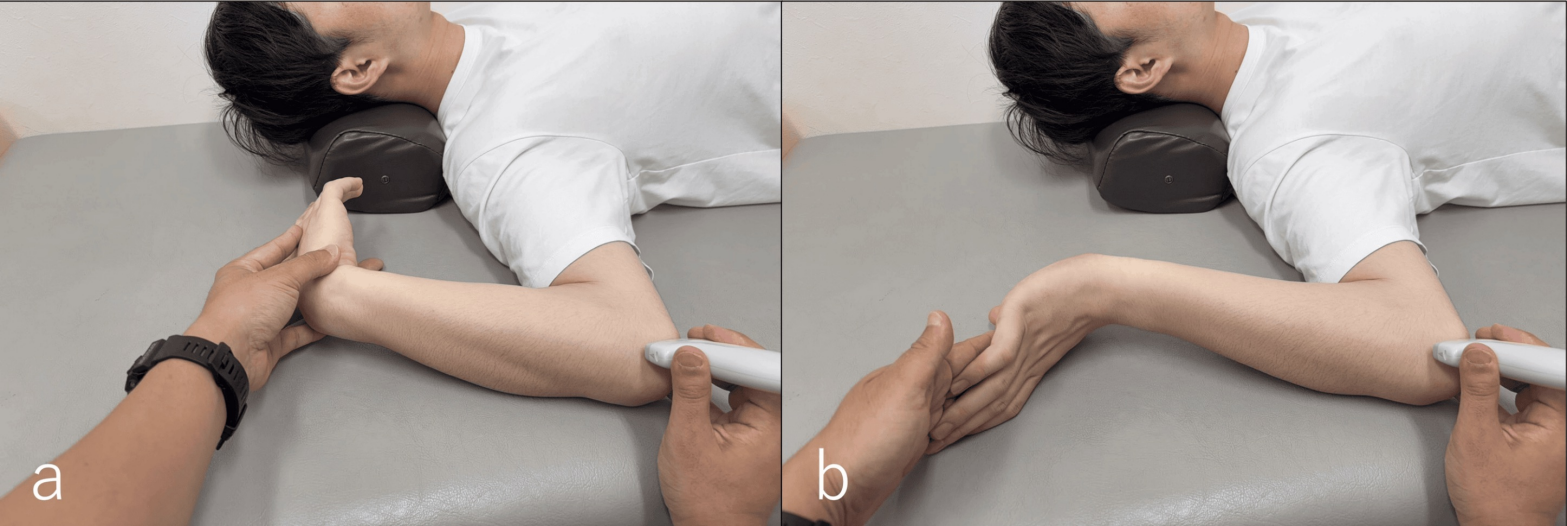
Ultrasound-guided UN sliding technique With the ulnar nerve imaged in the long axis at the cubital tunnel, sliding was induced in the pain-free range. (a) Wrist flexion with the elbow flexed and the forearm supinated. (b) Wrist extension with the elbow flexed and the forearm supinated. UN: ulnar nerve
